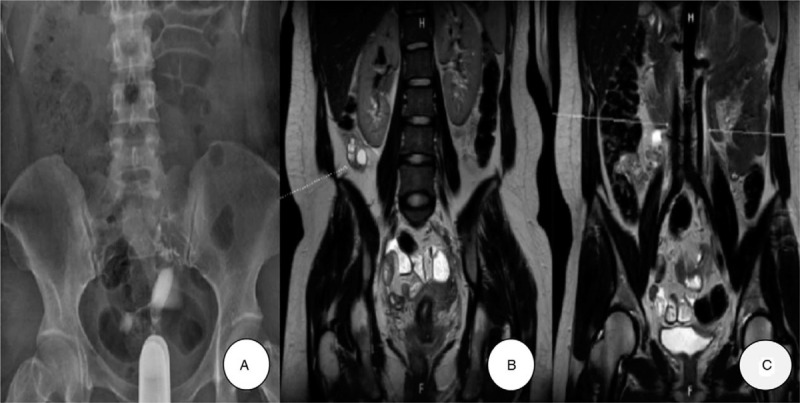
Postoperative hysterosalpingography (HSG) and magnetic resonance imaging (MRI) findings. (A) HSG showed a unicornuate uterus, preserved patency of left salpinx in the upper pole and some right salpinx in the lower pole. (B) MRI showed the right ovary at the right inferior hepatic portion (arrow). (C) MRI showed bilateral normal kidneys, and a double inferior vena cava (arrow).
